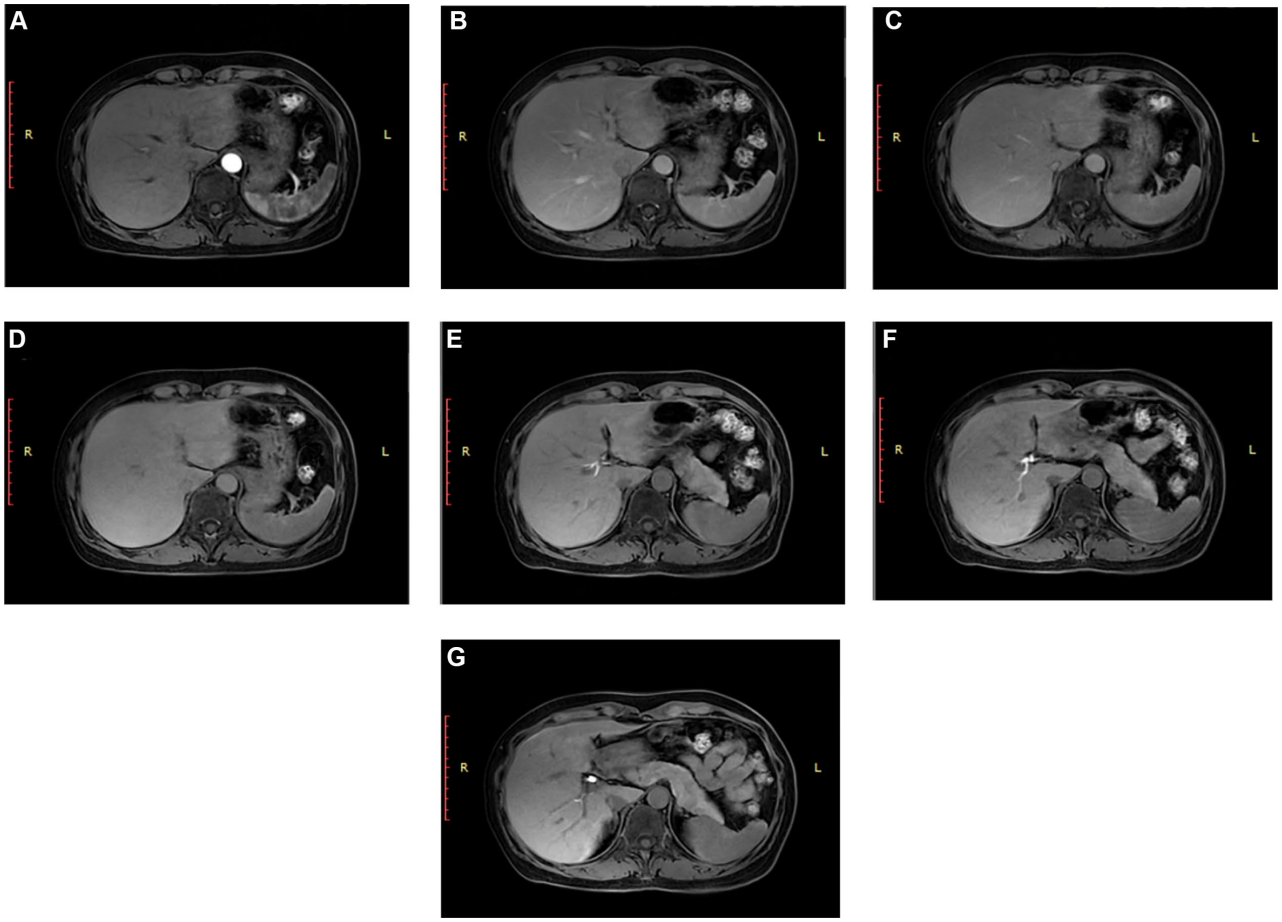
Gd-EOB-DTPA-enhanced hepatobiliary MRI. (A) Arterial phase, (B) portal venous phase, (C) delayed phase; (D) transitional phase, (E) hepatobiliary phase (20-min post-contrast), (F) hepatobiliary phase (25-min post-contrast), and (G) hepatobiliary phase (30-min post-contrast).
